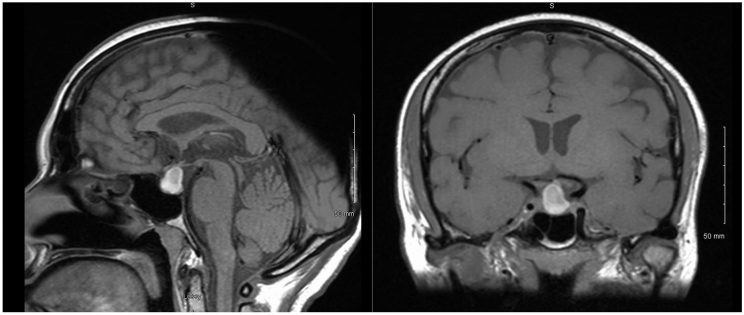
Sagittal and coronal views of T1-weighted images of pituitary magnetic resonance imaging showing an expansile sellar and suprasellar hyperintense lesion measuring 13.5 × 9 × 11 mm.
